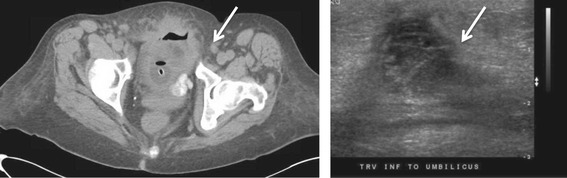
CT scan (left) and Ultrasound (right) of the pelvis. These studies demonstrate a smaller, heterogeneous mass (arrows) with a solid superior compartment and a walled-off, phlegmon-filled inferior compartment.
